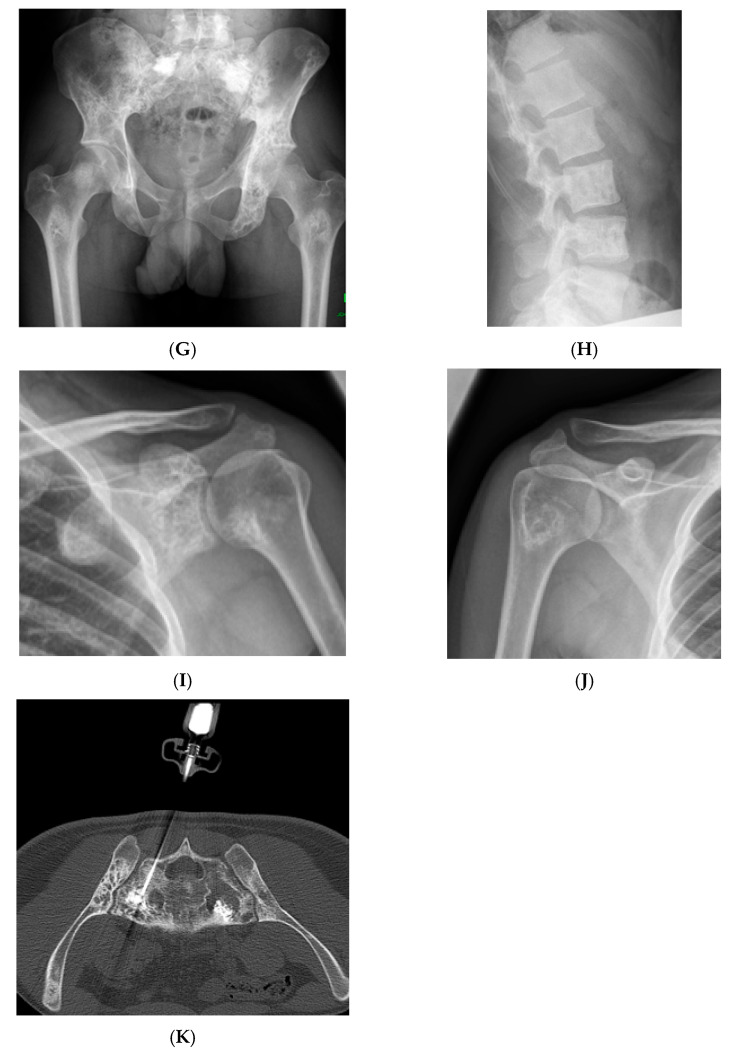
Multiple haemangiomas. (A) Coronal T1W MRI whole body with side-by-side coronal STIR MRI whole body showing multiple haemangiomas at the vertebral column, pelvis, and proximal femora. (B) Axial T1W Pelvis MRI and (C) STIR Pelvis MRI, further detailing the pelvis lesions. (D) Sagittal T1W Spine MRI with spine haemangiomas. (E) Sagittal T2W Spine MRI with spine haemangiomas. (F) Sagittal STIR Spine MRI with spine haemangiomas. (G) Plain radiograph, pelvis. (H) Plain radiograph, lumbar spine. (I) Plain radiograph, left shoulder. (J) Plain radiograph, right shoulder. All radiographs showcase the corresponding appearances of the lesion on plain films. (K) Axial CT pelvis with biopsy-proven haemangioma at the right hemi-pelvis.
